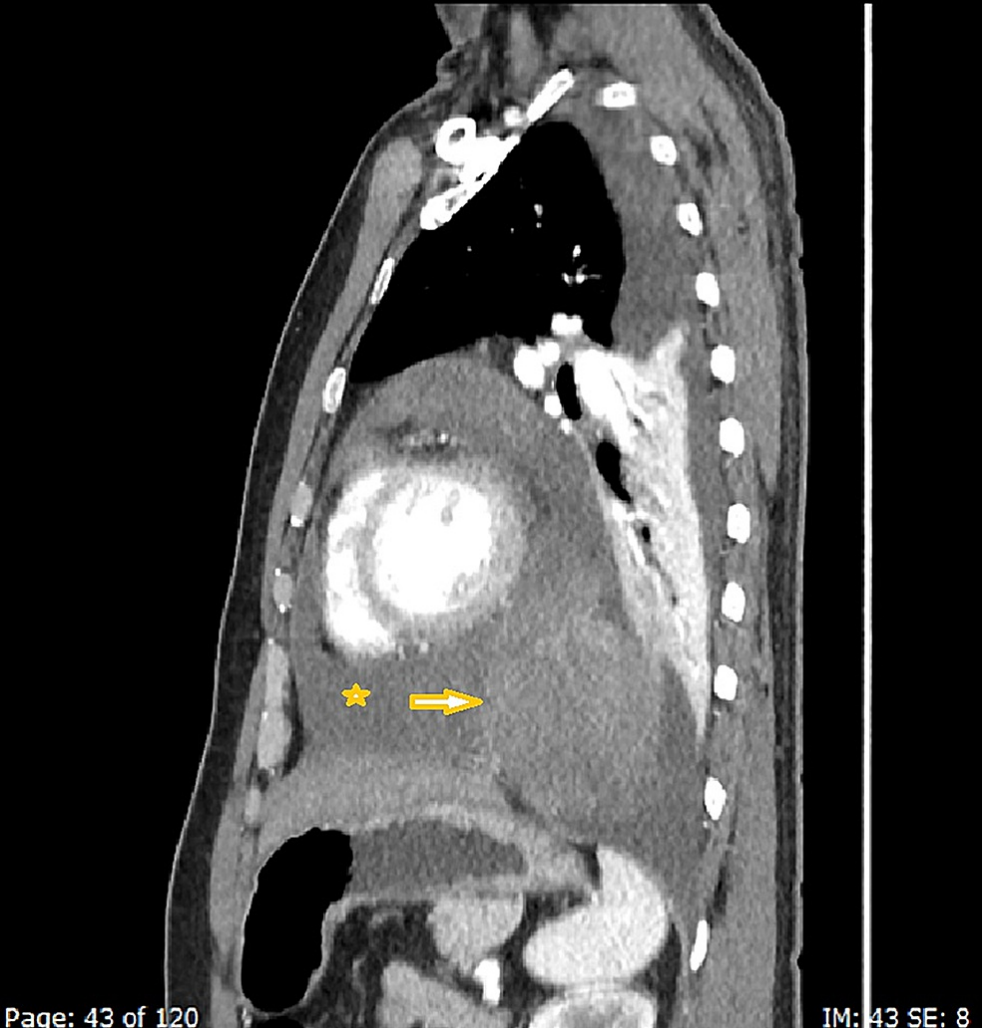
Sagittal contrast CT (arterial phase) images showing a large, heterogeneously enhancing pericardial mass (arrowhead) with pericardial effusion (star)
